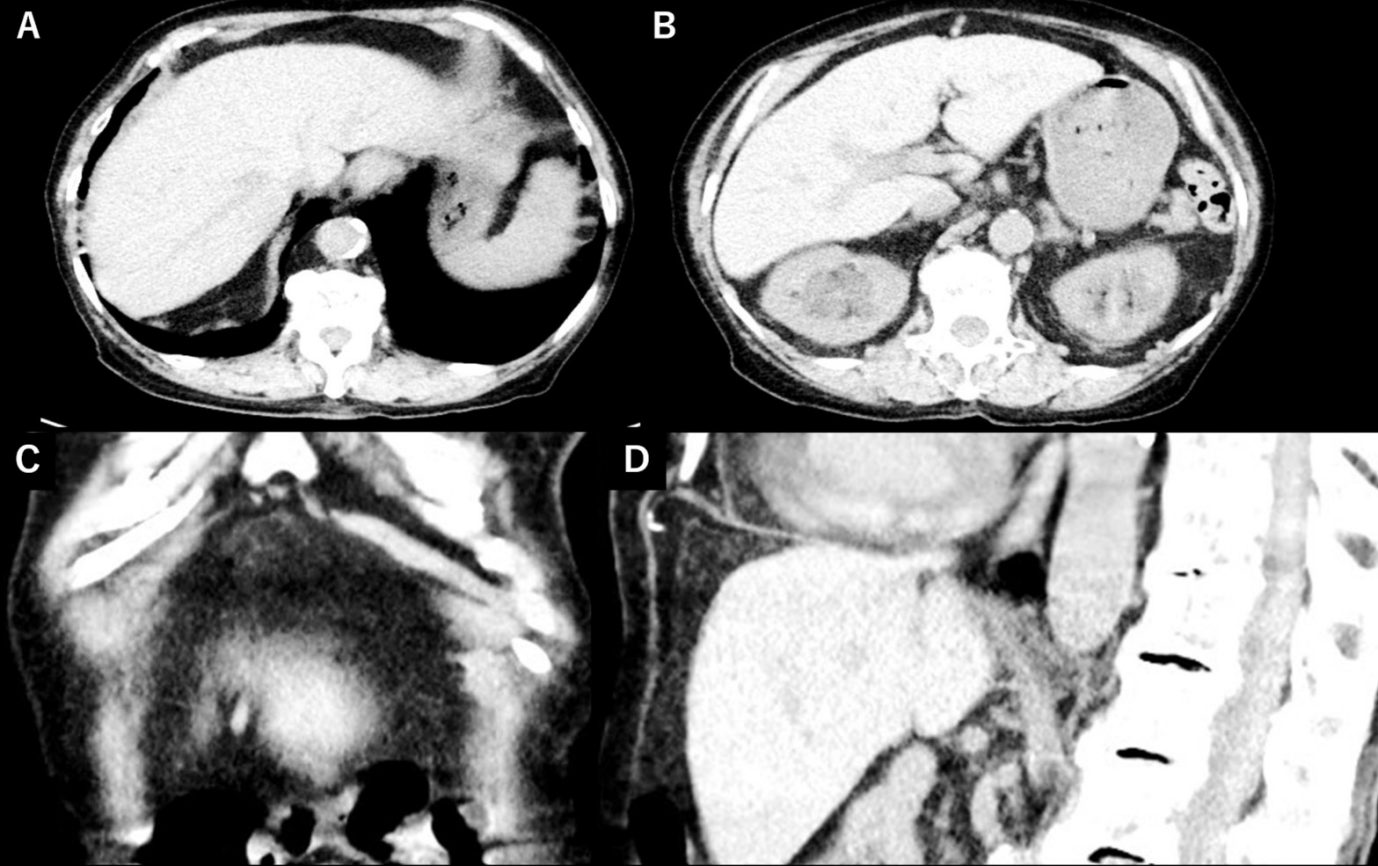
Follow‐up CT images obtained the day after admission, corresponding to the same anatomical planes as in Figure 1B–E. (A, B) Axial, (C) coronal, and (D) sagittal abdominal‐window CT images show complete resolution of the previously noted intraperitoneal free air. These findings parallel the patient's clinical improvement following conservative treatment.
